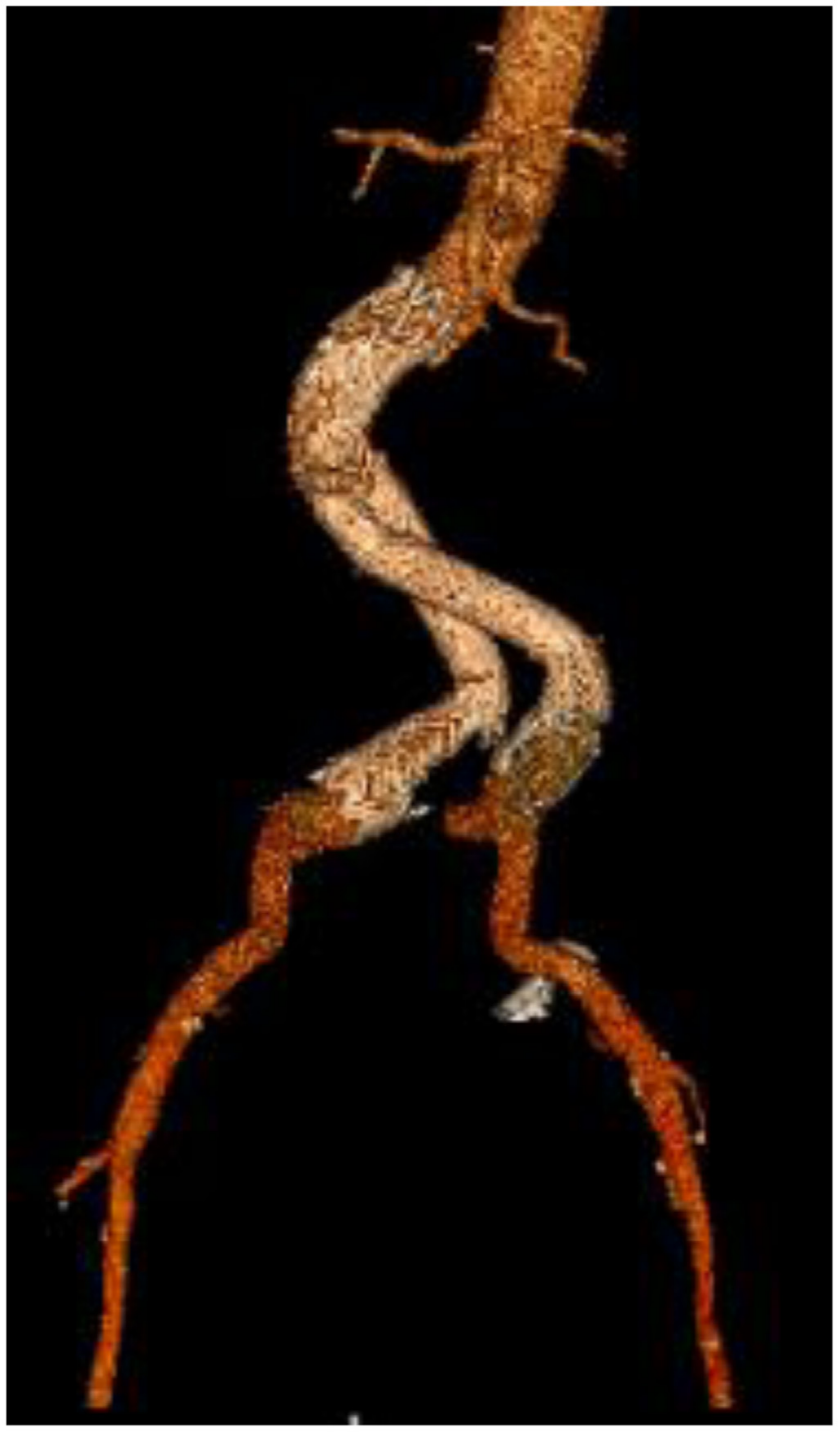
Computed tomography (*CT*) reconstruction of endograft at 1-year surveillance appointment.
